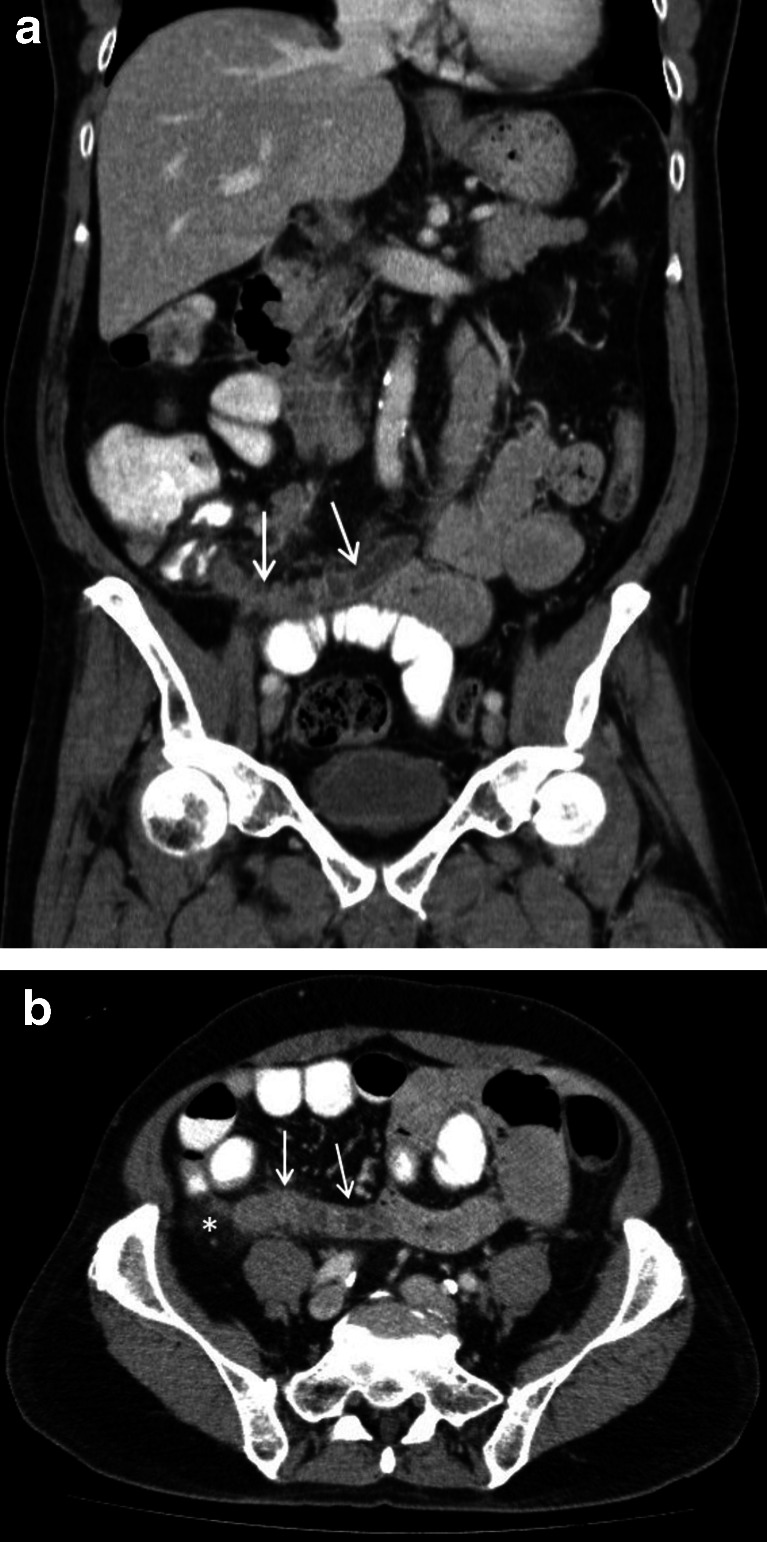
A 64-year-old male with moderately differentiated adenocarcinoma of the appendix (case 4). The coronal (a) and axial (b) contrast-enhanced CT scan images reveal a dilated, fluid-filled appendix with mural thickening and enhancement (*white arrows*). There is also evidence of periappendiceal inflammatory fat stranding (*white asterisk*) which is best appreciated in the axial image
